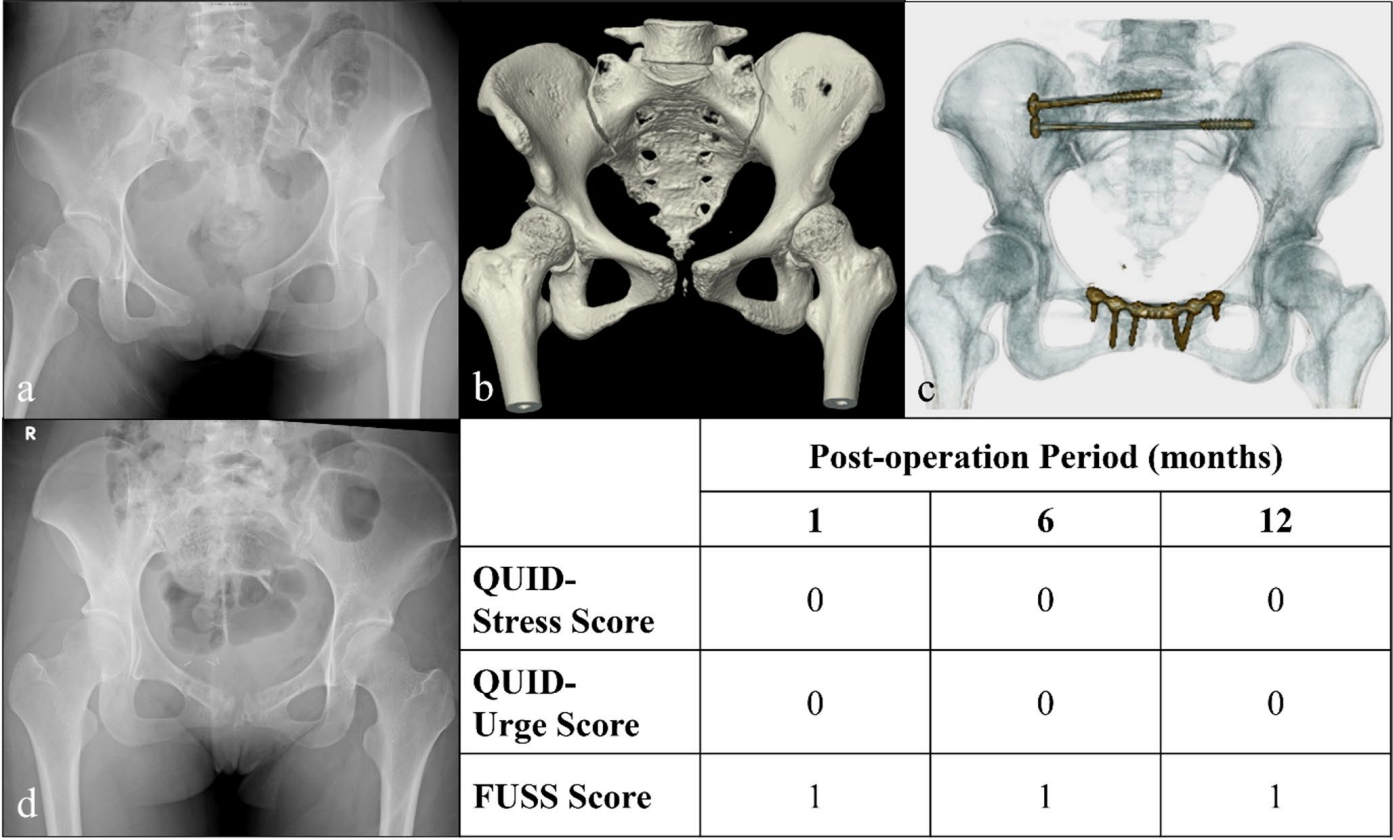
Case presentation of a 21-year-old woman with a PRI classified as APC II (AO B2.3) sustained in a motor vehicle accident with related urinary sequelae assessments. (a) and (b) Preoperative X-ray (without pelvic binder) and CT scan (with pelvic binder). (c) Postoperative CT image. (d) One-year postoperative follow-up image
